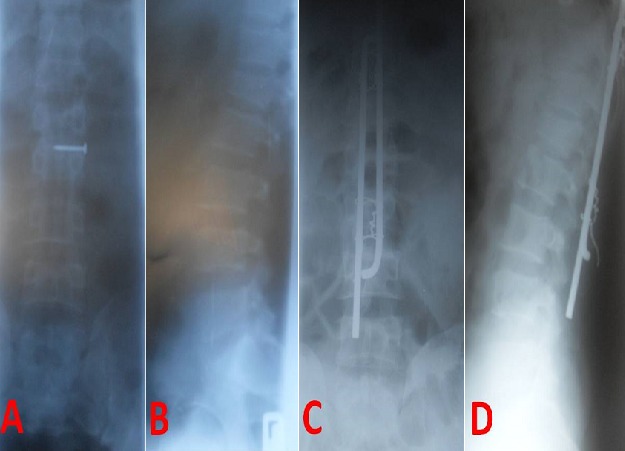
Pre- (A, B) and post-operative (C, D) X-rays in a trauma patient with L1 compression fracture. The nail over L1 spinous process (A) was used for pre-operative spinal marking. D shows slight rotation of one vertical strut.
